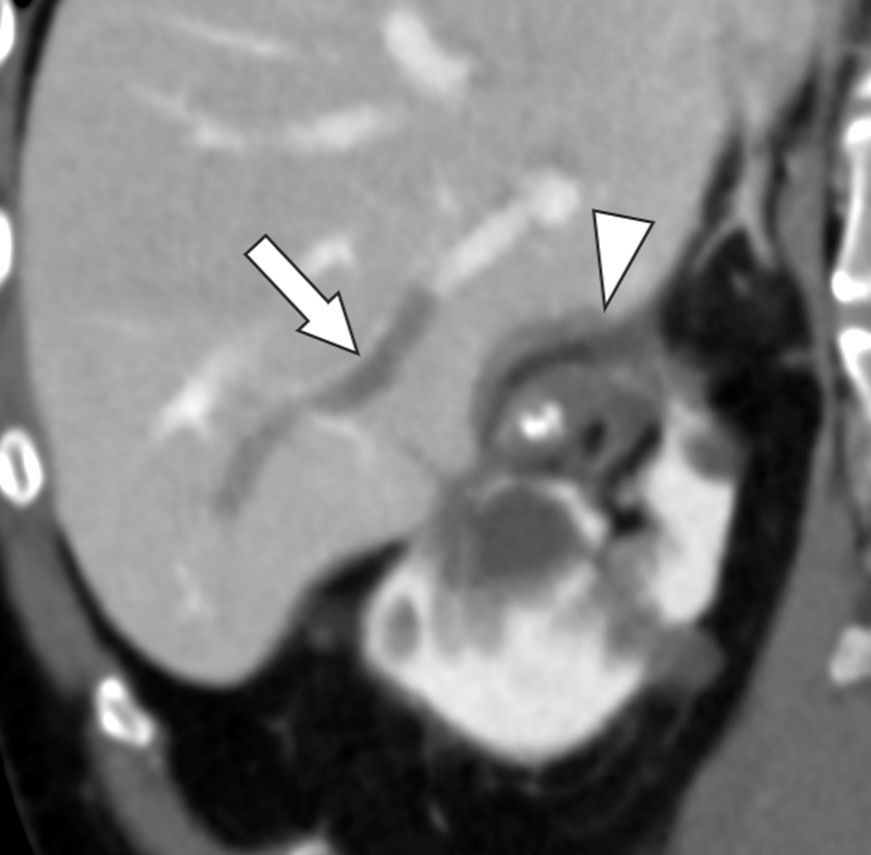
Oblique coronal contrast-enhanced CT 2 days after cryoablation reveals the ablated liver parenchyma (arrowhead) and portal venous thrombus (arrow).
